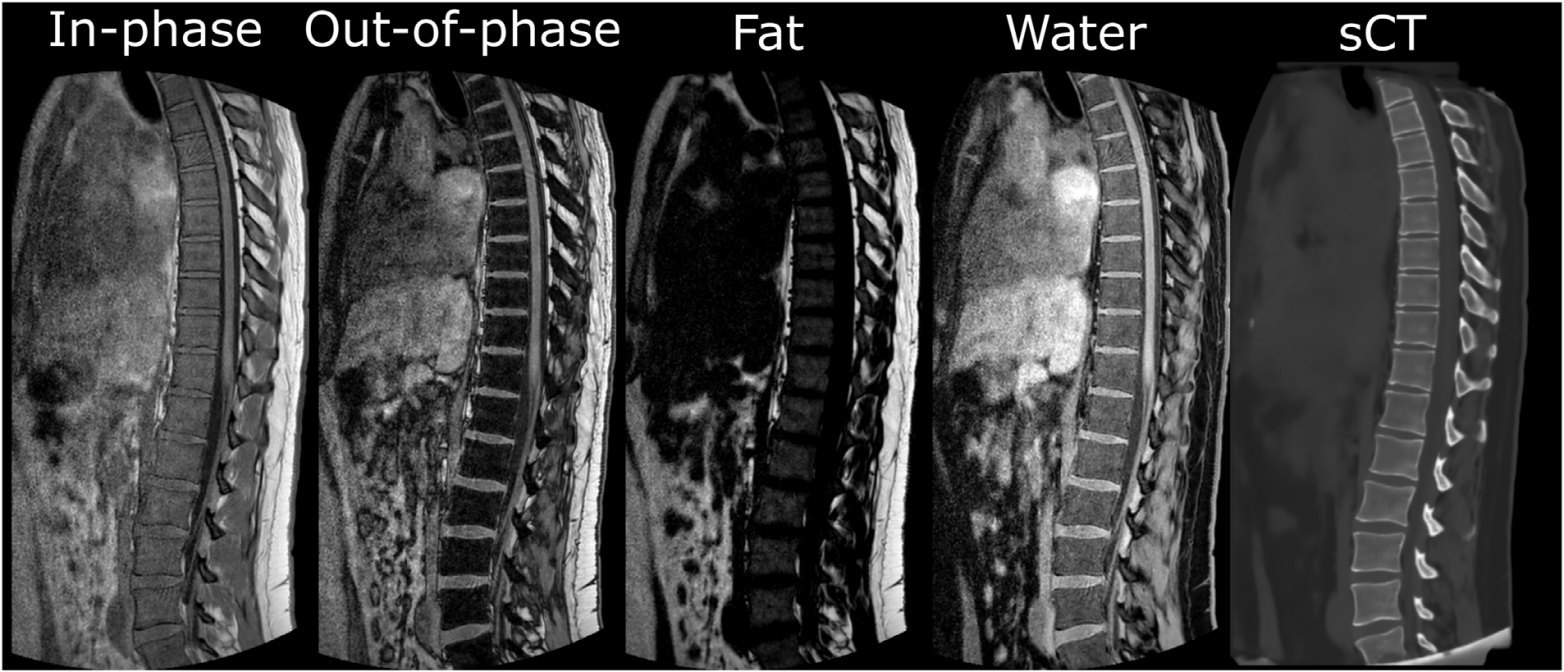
Sagittal slices from the 3D in- and out-of-phase images, the fat and water reconstructed images, and the generated synthetic CT images.
